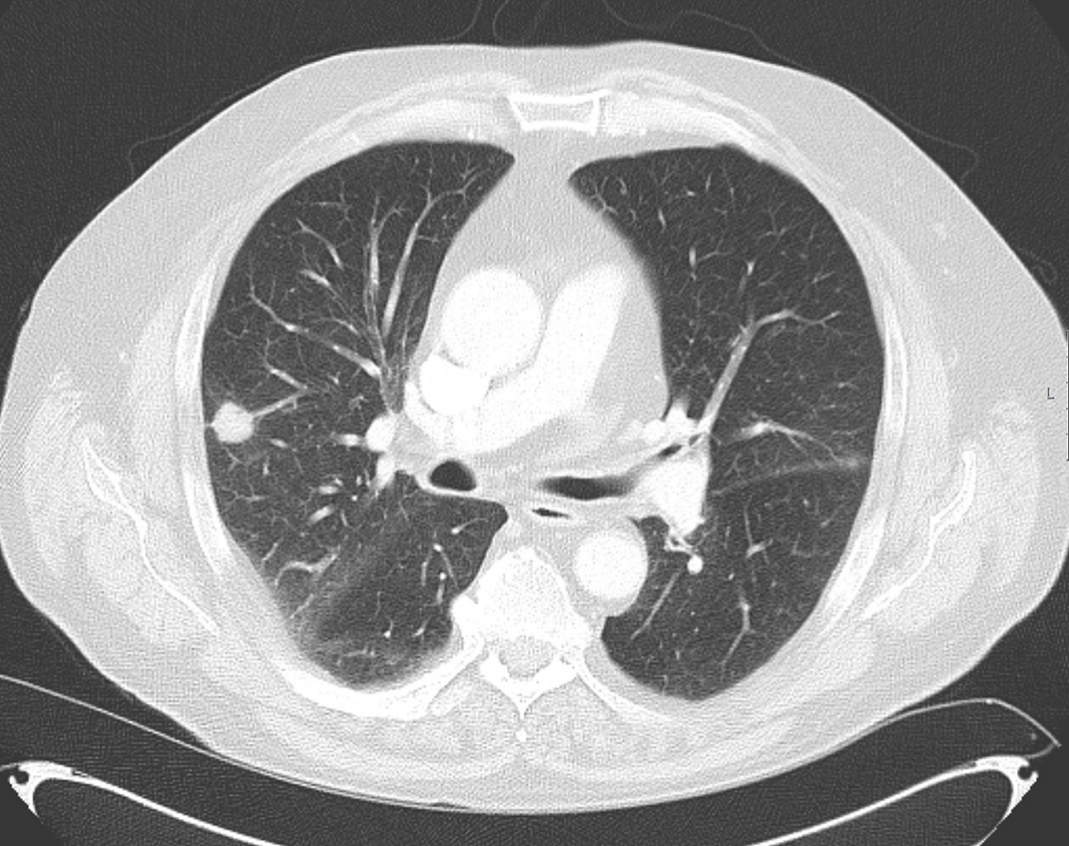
Axial lung window reformat of original diagnostic CT image demonstrating a small soft tissue nodule in the right upper lobe.
